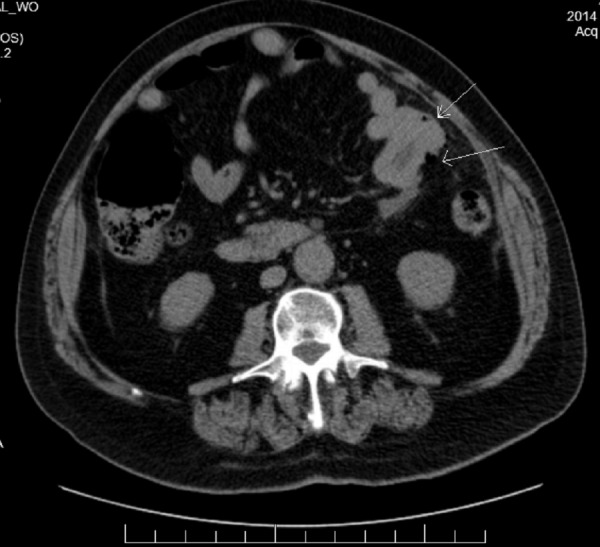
CT of abdomen showing evidence of thickened bowel as pointed with white arrows, along with pockets of free peritoneal air.
